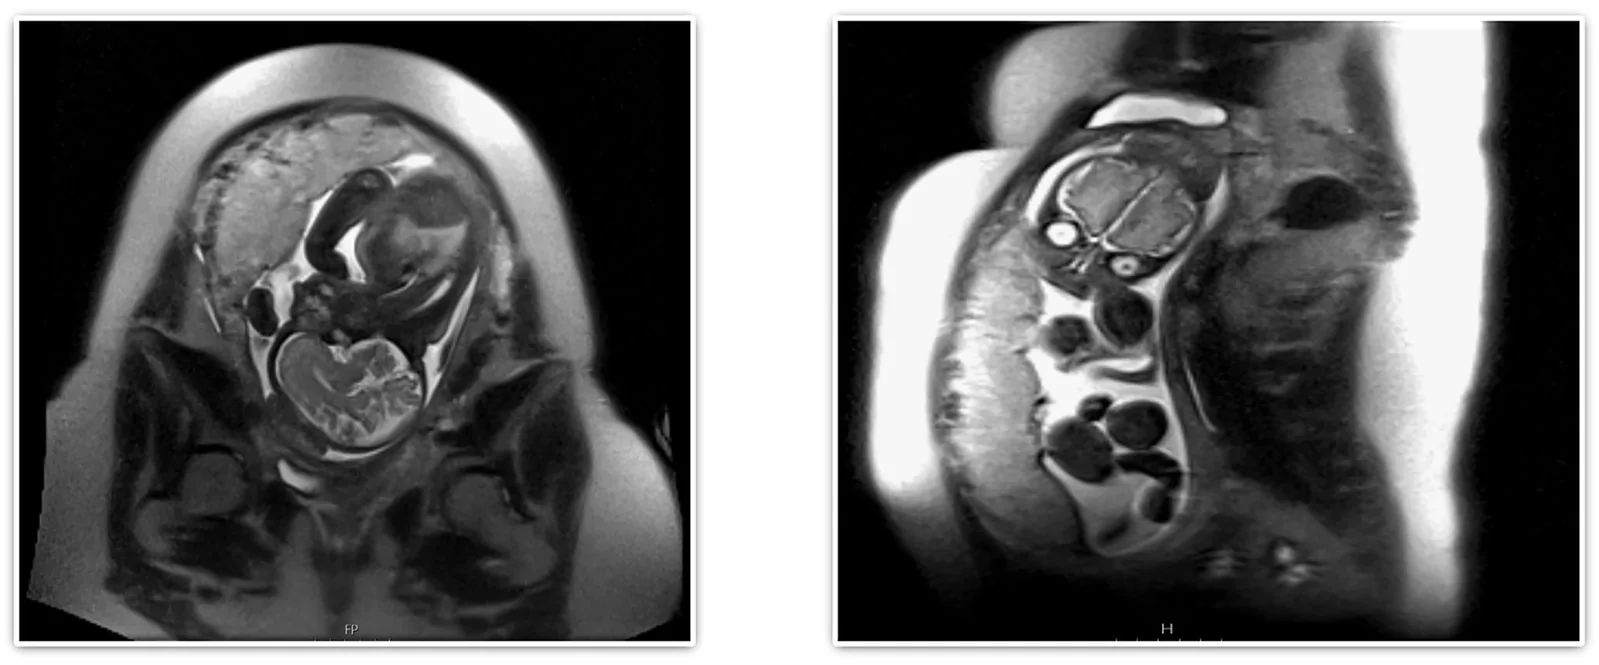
Magnetic resonance images of glomovenous malformation (GVM) on sagittal and coronal views of T2 weighted images demonstrating the scalp soft tissue mass with no cystic changes, no obvious blood vessels and no underlying skull defect.
